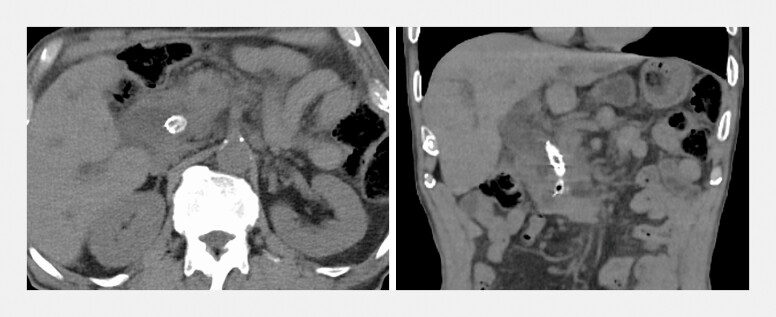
Computed tomography image showed dilation of the bile duct due to the stent occlusion.
